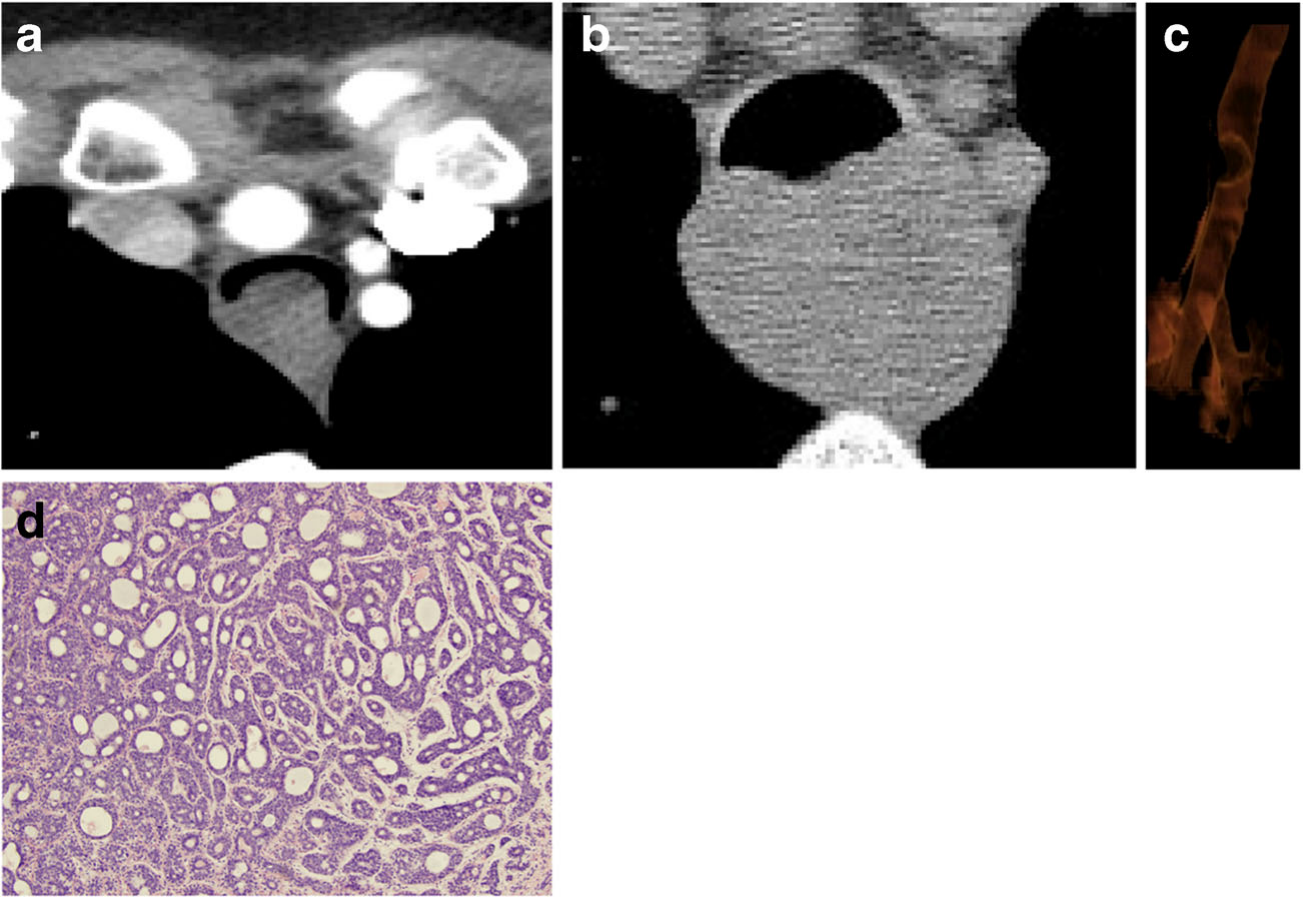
Fifty-year-old woman with a 1-year history of dyspnoea on exertion. Axial a contrast and b non-contrast CT images demonstrating a large, eccentric soft tissue mass within the trachea. Image b demonstrates a large mass with obliteration of the fat plane with the oesophagus. c 3D VR image shows significant focal narrowing by the posterior mass with endoluminal extension. d Pathology reveals cribriform and microcystic growth patterns of bland basaloid cells with scant cytoplasm and angulated, hyperchromatic nuclei compatible with adenoid cystic carcinoma
